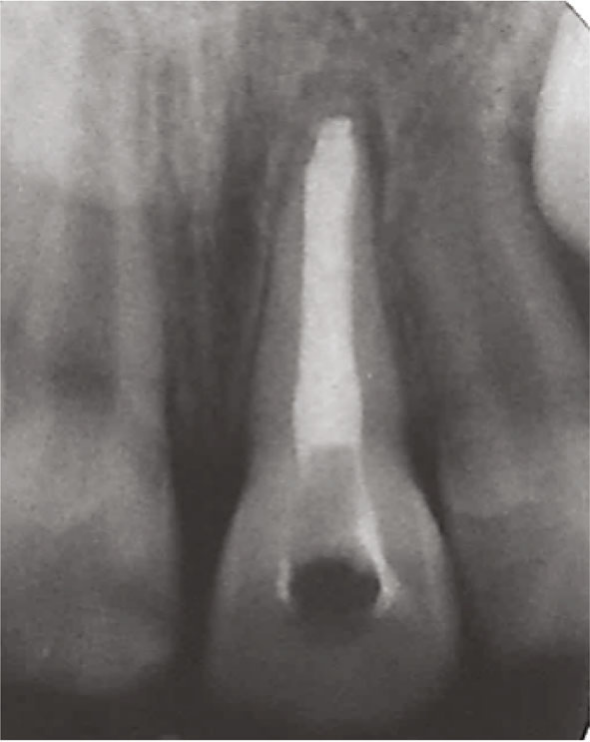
Posteroanterior radiograph after obturating with MTA (6 months after initial root canal treatment).
